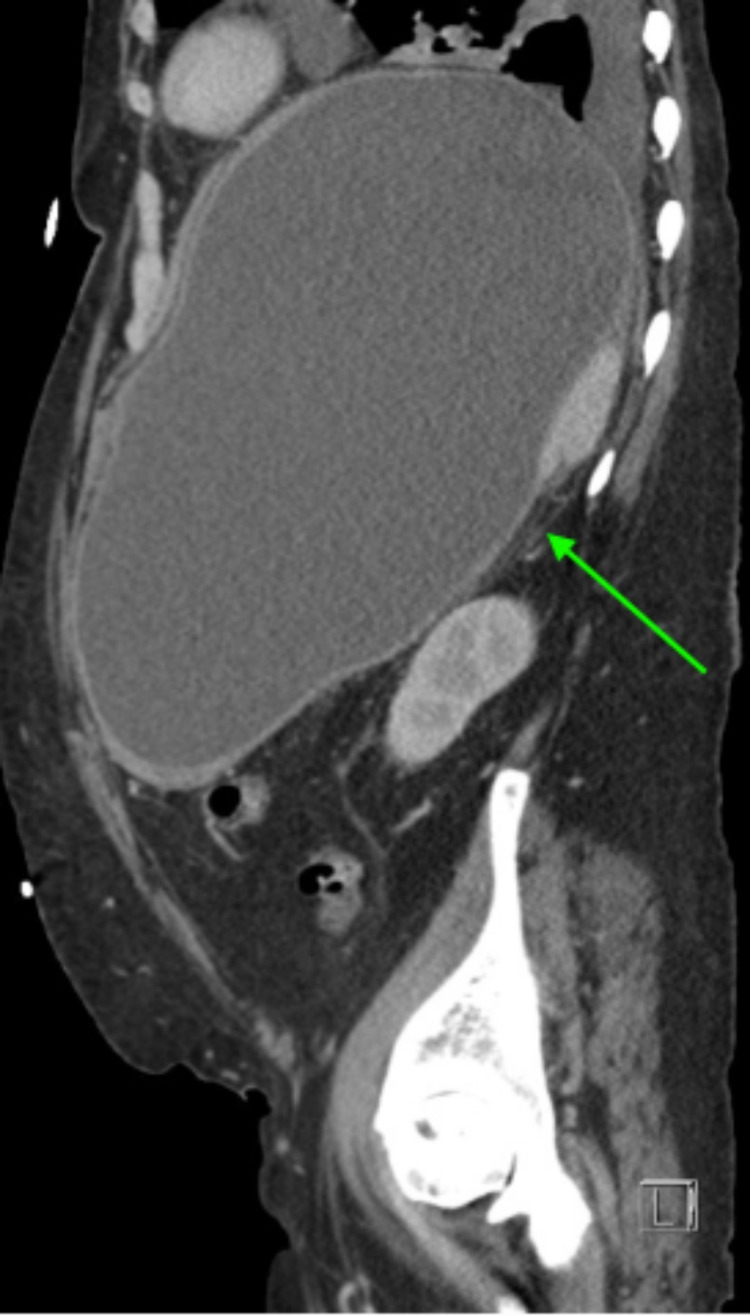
Sagittal view CT of the abdomen and pelvis with intravenous contrast demonstrating a large fluid collection in the left upper quadrant (arrow). CT - computed tomography
